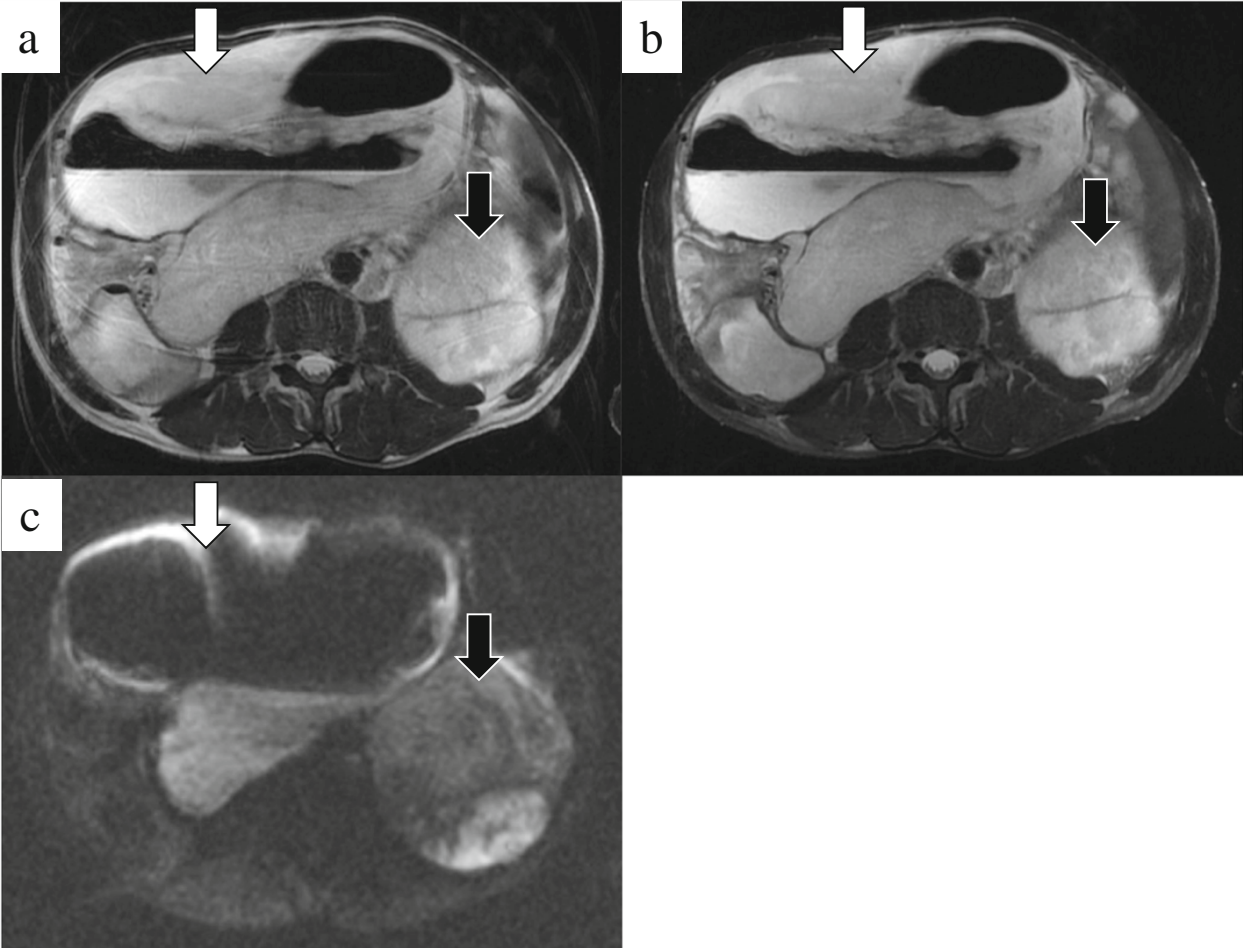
Non-contrast abdominal magnetic resonance image. a The solid part of both intra-abdominal (white arrow) and retroperitoneal (black arrow) masses showed inhomogeneously high intensity in T2-weighted imaging (T2WI). b Both masses also showed almost the same pattern as T2WI in fat saturation T2WI. c Both masses also showed inhomogeneously high intensity in diffusion-weighted imaging
